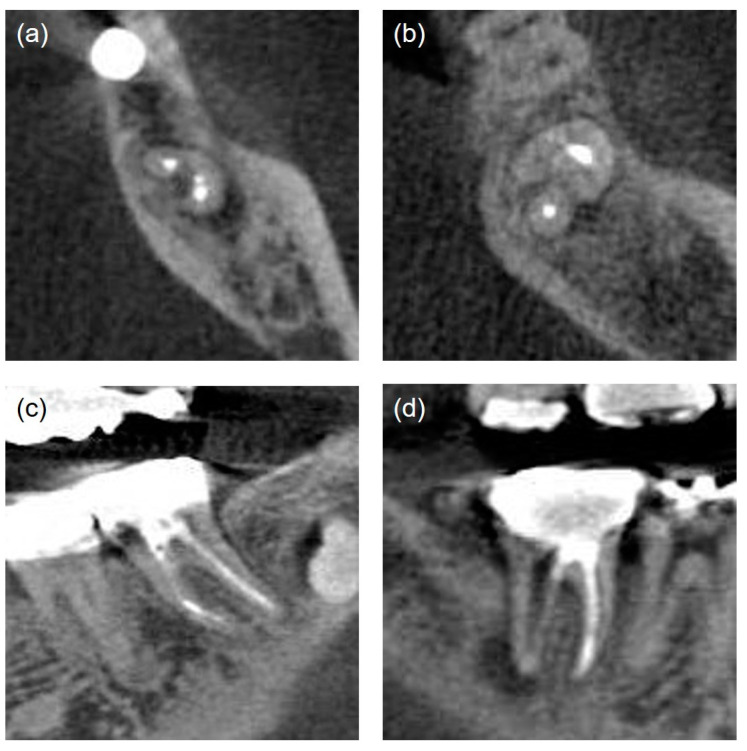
The quality of root canal treatment was evaluated by analyzing CBCT cross-sectional images. (a) Untreated isthmus area of the C-shaped canal in the mandibular right second molar, axial view. (b) Missed mesio-lingual canal in the mandibular right second molar, axial view. (c) Instrument fracture in the mesial root canal of the mandibular left second molar, coronal view. (d) Perforation in the furcation area of the mandibular right second molar, coronal view.
